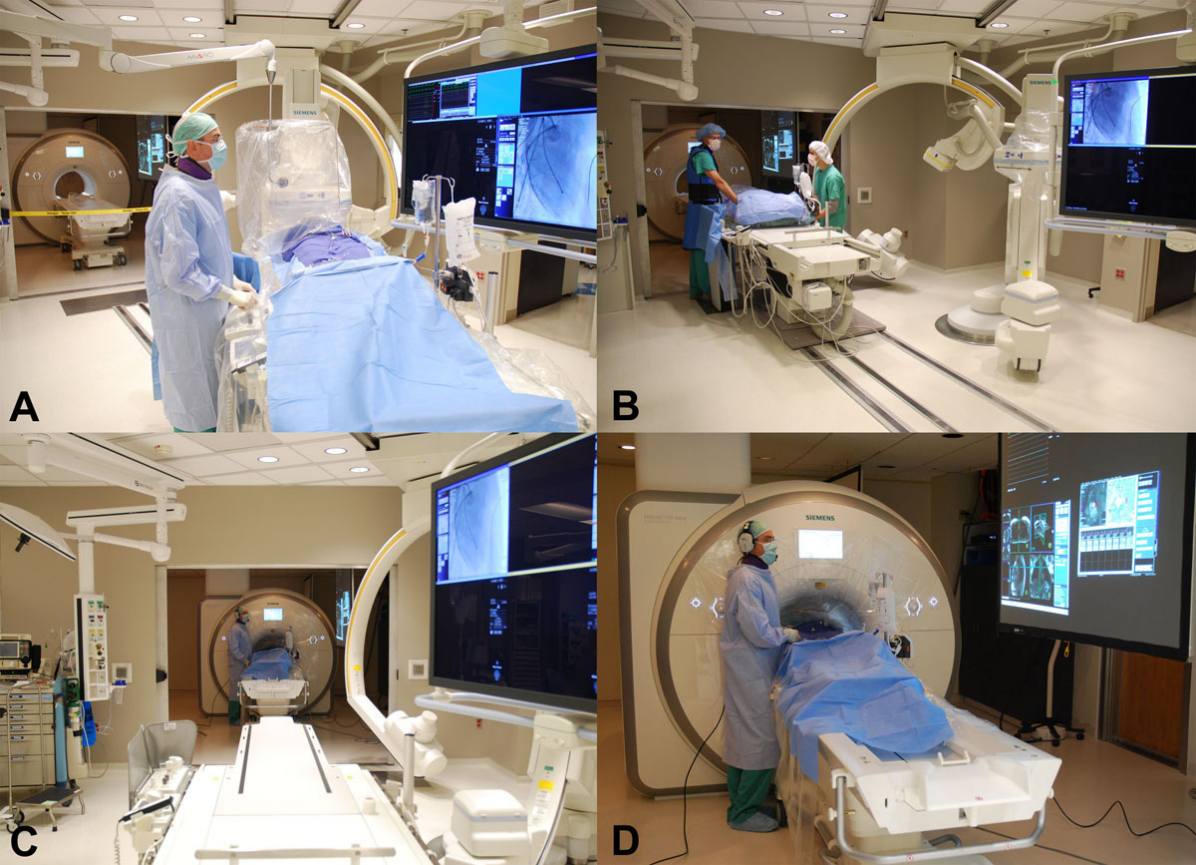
(A) If required X-ray coronary angiography is performed before MR RHC. (B) The patient is transferred to MRI maintaining sterility of the field. (C) View from X-ray into the MR room. (D) MR RHC performed from the femoral vein. Images are projected onto a screen for the operator. Noise-suppression headsets are used for communication between staff and with the patient.
